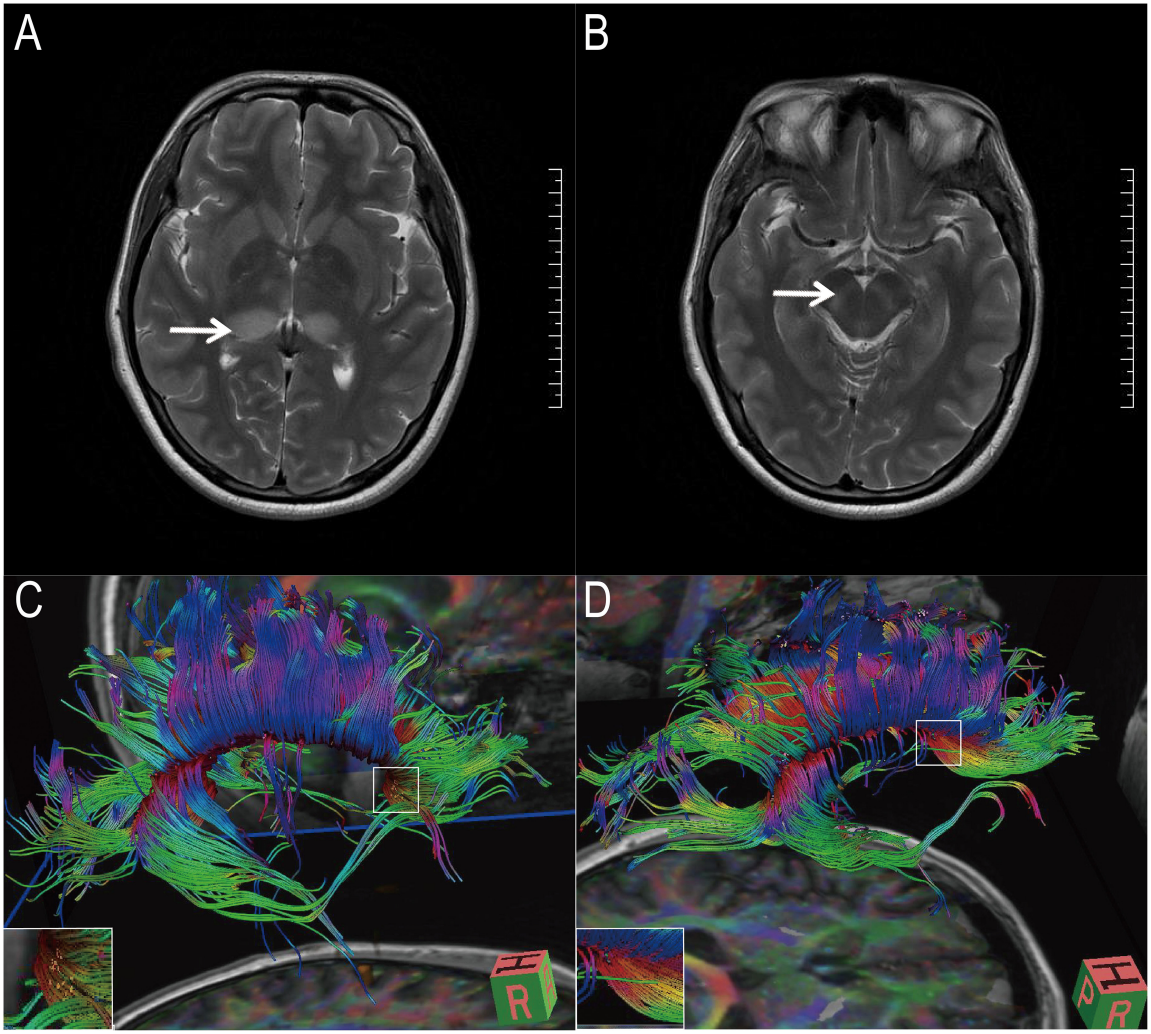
Magnetic resonance image and diffusion tensor imaging image of 1 patient. (A,B) Magnetic resonance images showed that the patient's thalamus (white arrow) and basal ganglia (white arrow) were lesions. (C,D) Diffusion tensor imaging showed that the patient's corpus callosum tract was partially broken. Three months later, the patient's corpus callosum rupture improved.
